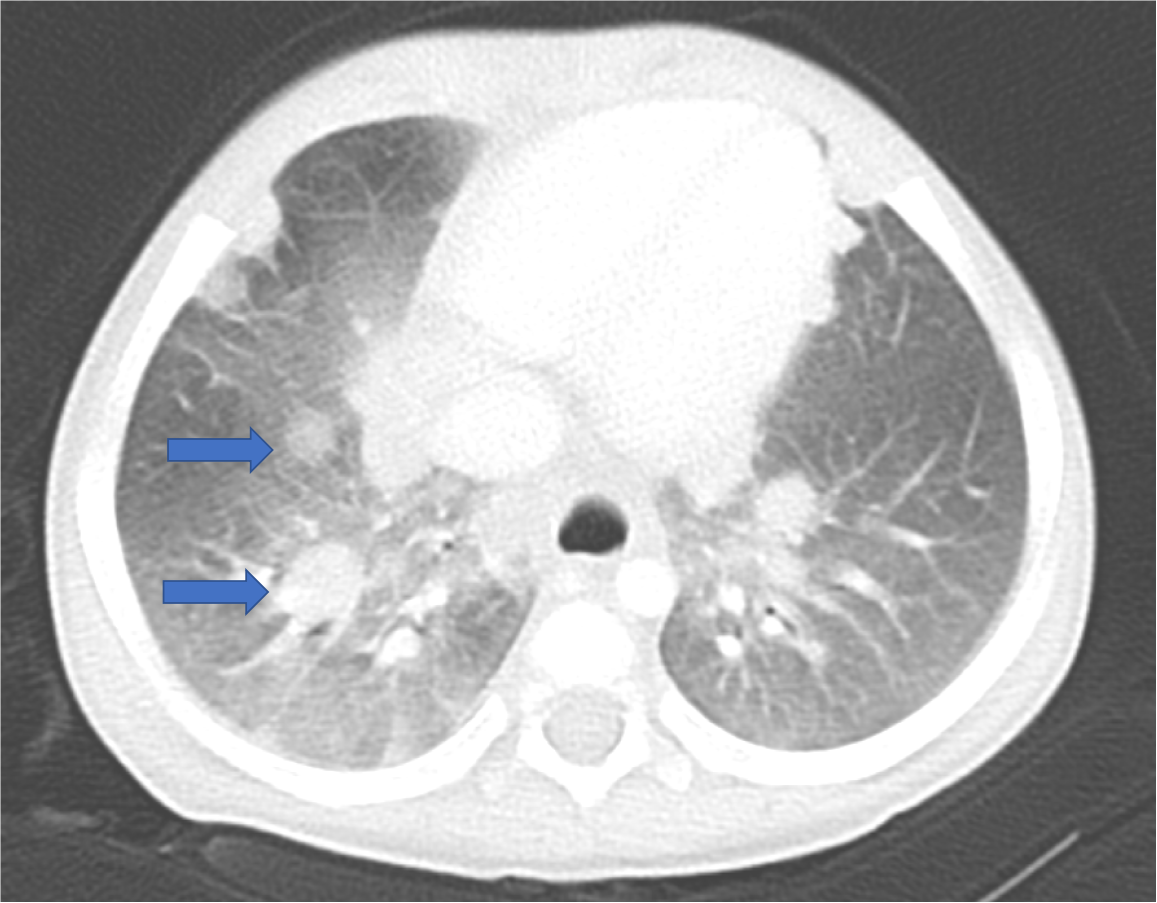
Multiple lung nodules. Contrast‐enhanced computed tomography showed multiple lung nodules (arrows) that were considered for lung biopsy, but a biopsy was not performed due to the patient's severe respiratory insufficiency.
